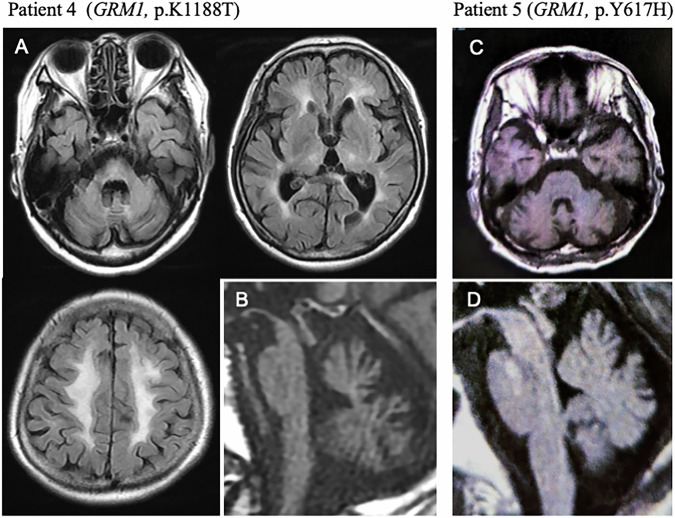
Head images of the patients with GRM1 variants. A Axial fluid-attenuated inversion recovery images of Patient 4 show cerebellar and brainstem atrophy and white matter lesions in the periventricular and subcortical regions. B Sagittal T1-weighted image of Patient 5, showing cerebellar and brainstem atrophy. Axial (C) and sagittal (D) T1-weighted images of Patient 5 showed cerebellar and brainstem atrophy
